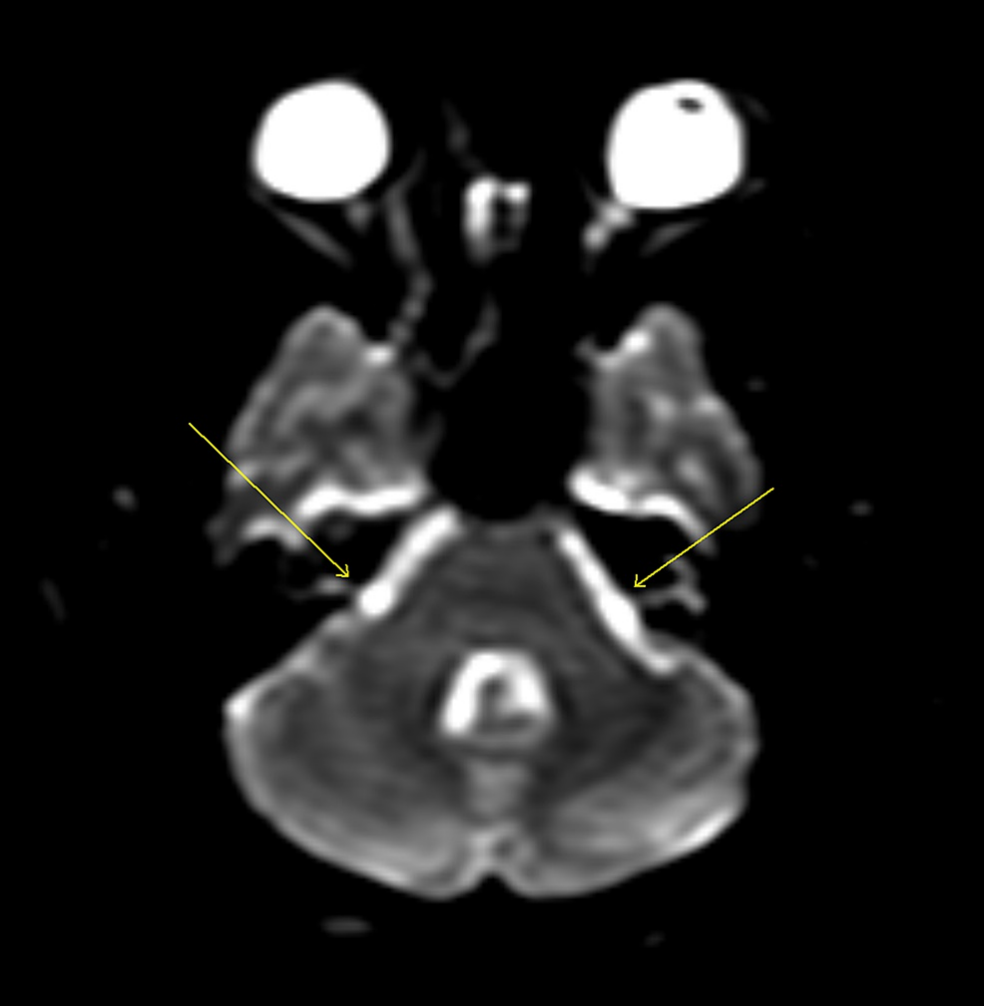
Diffusion-weighted MRI. The arrows show marked improvement in the brachium pontis lesion.
